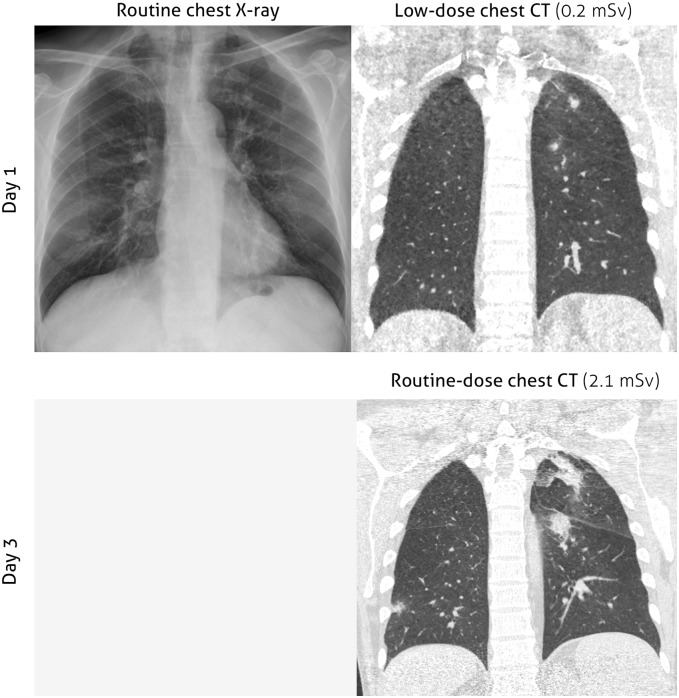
Patient with a positive LDCT scan for fungal infection on day 1 of neutropenic fever. Upper row left: CXR acquired on day 1 of febrile neutropenia without signs of pulmonary infection. Upper row right: LDCT images showing solid consolidations with halo signs suspect for IFD at day 1 of febrile neutropenia. Lower row: HRCT acquired on the 3^rd^ day of neutropenic fever shows progression of the consolidations and new consolidations.
